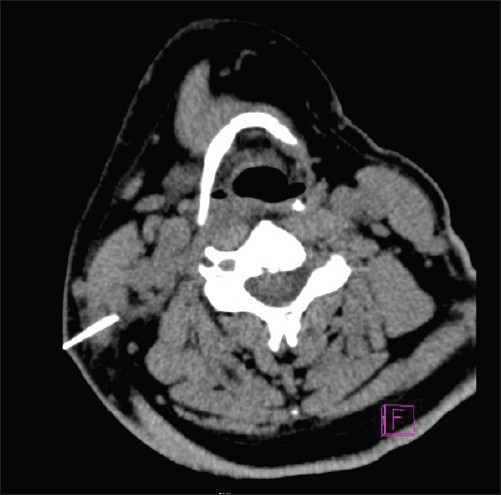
CT revealing the fish bone transversely located at the right sternocleidomastoid muscle.
